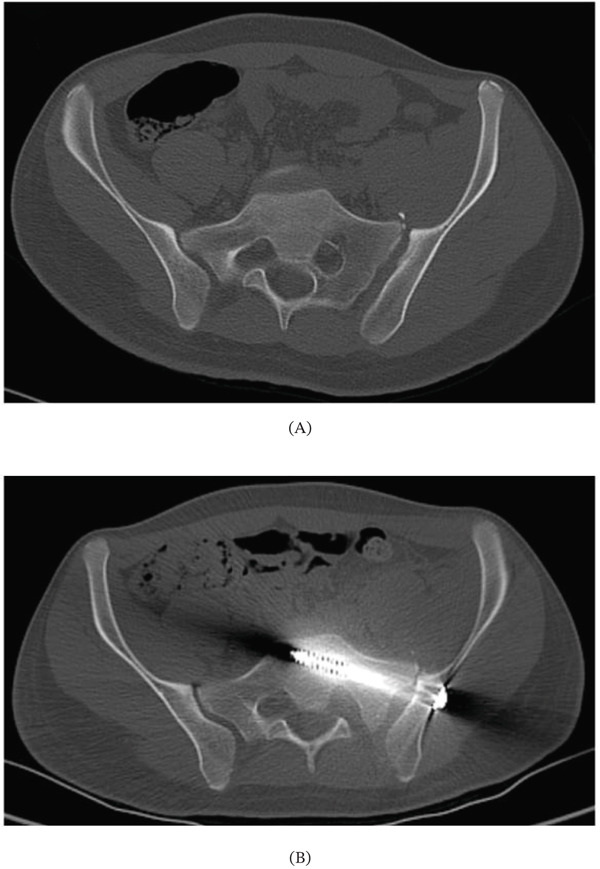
Postoperative CT scan. Comparative axial CT images of the upper sacral segment obtained (A) preoperatively and (B) after iliosacral fixation.
